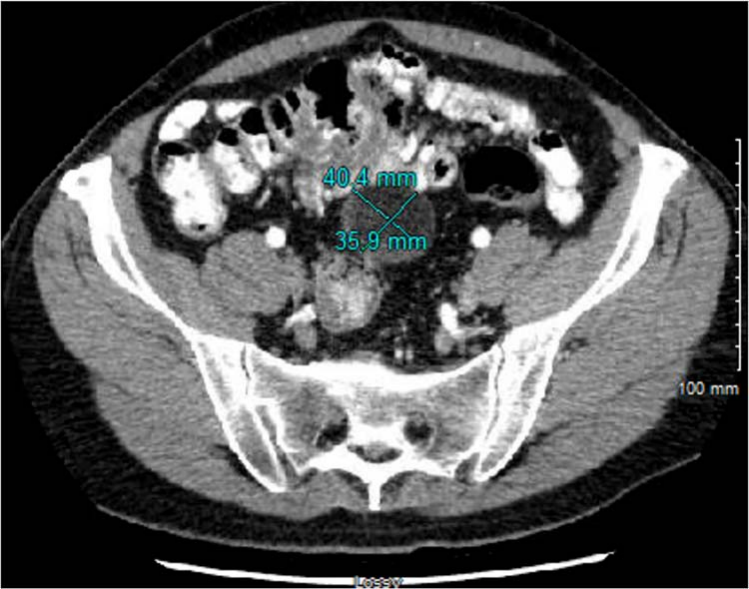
CT imaging from 4 months after initial diagnosis, showing a 4.0 × 3.6 × 3.8 cm, seemingly lipomatous mass in the midpelvis.
